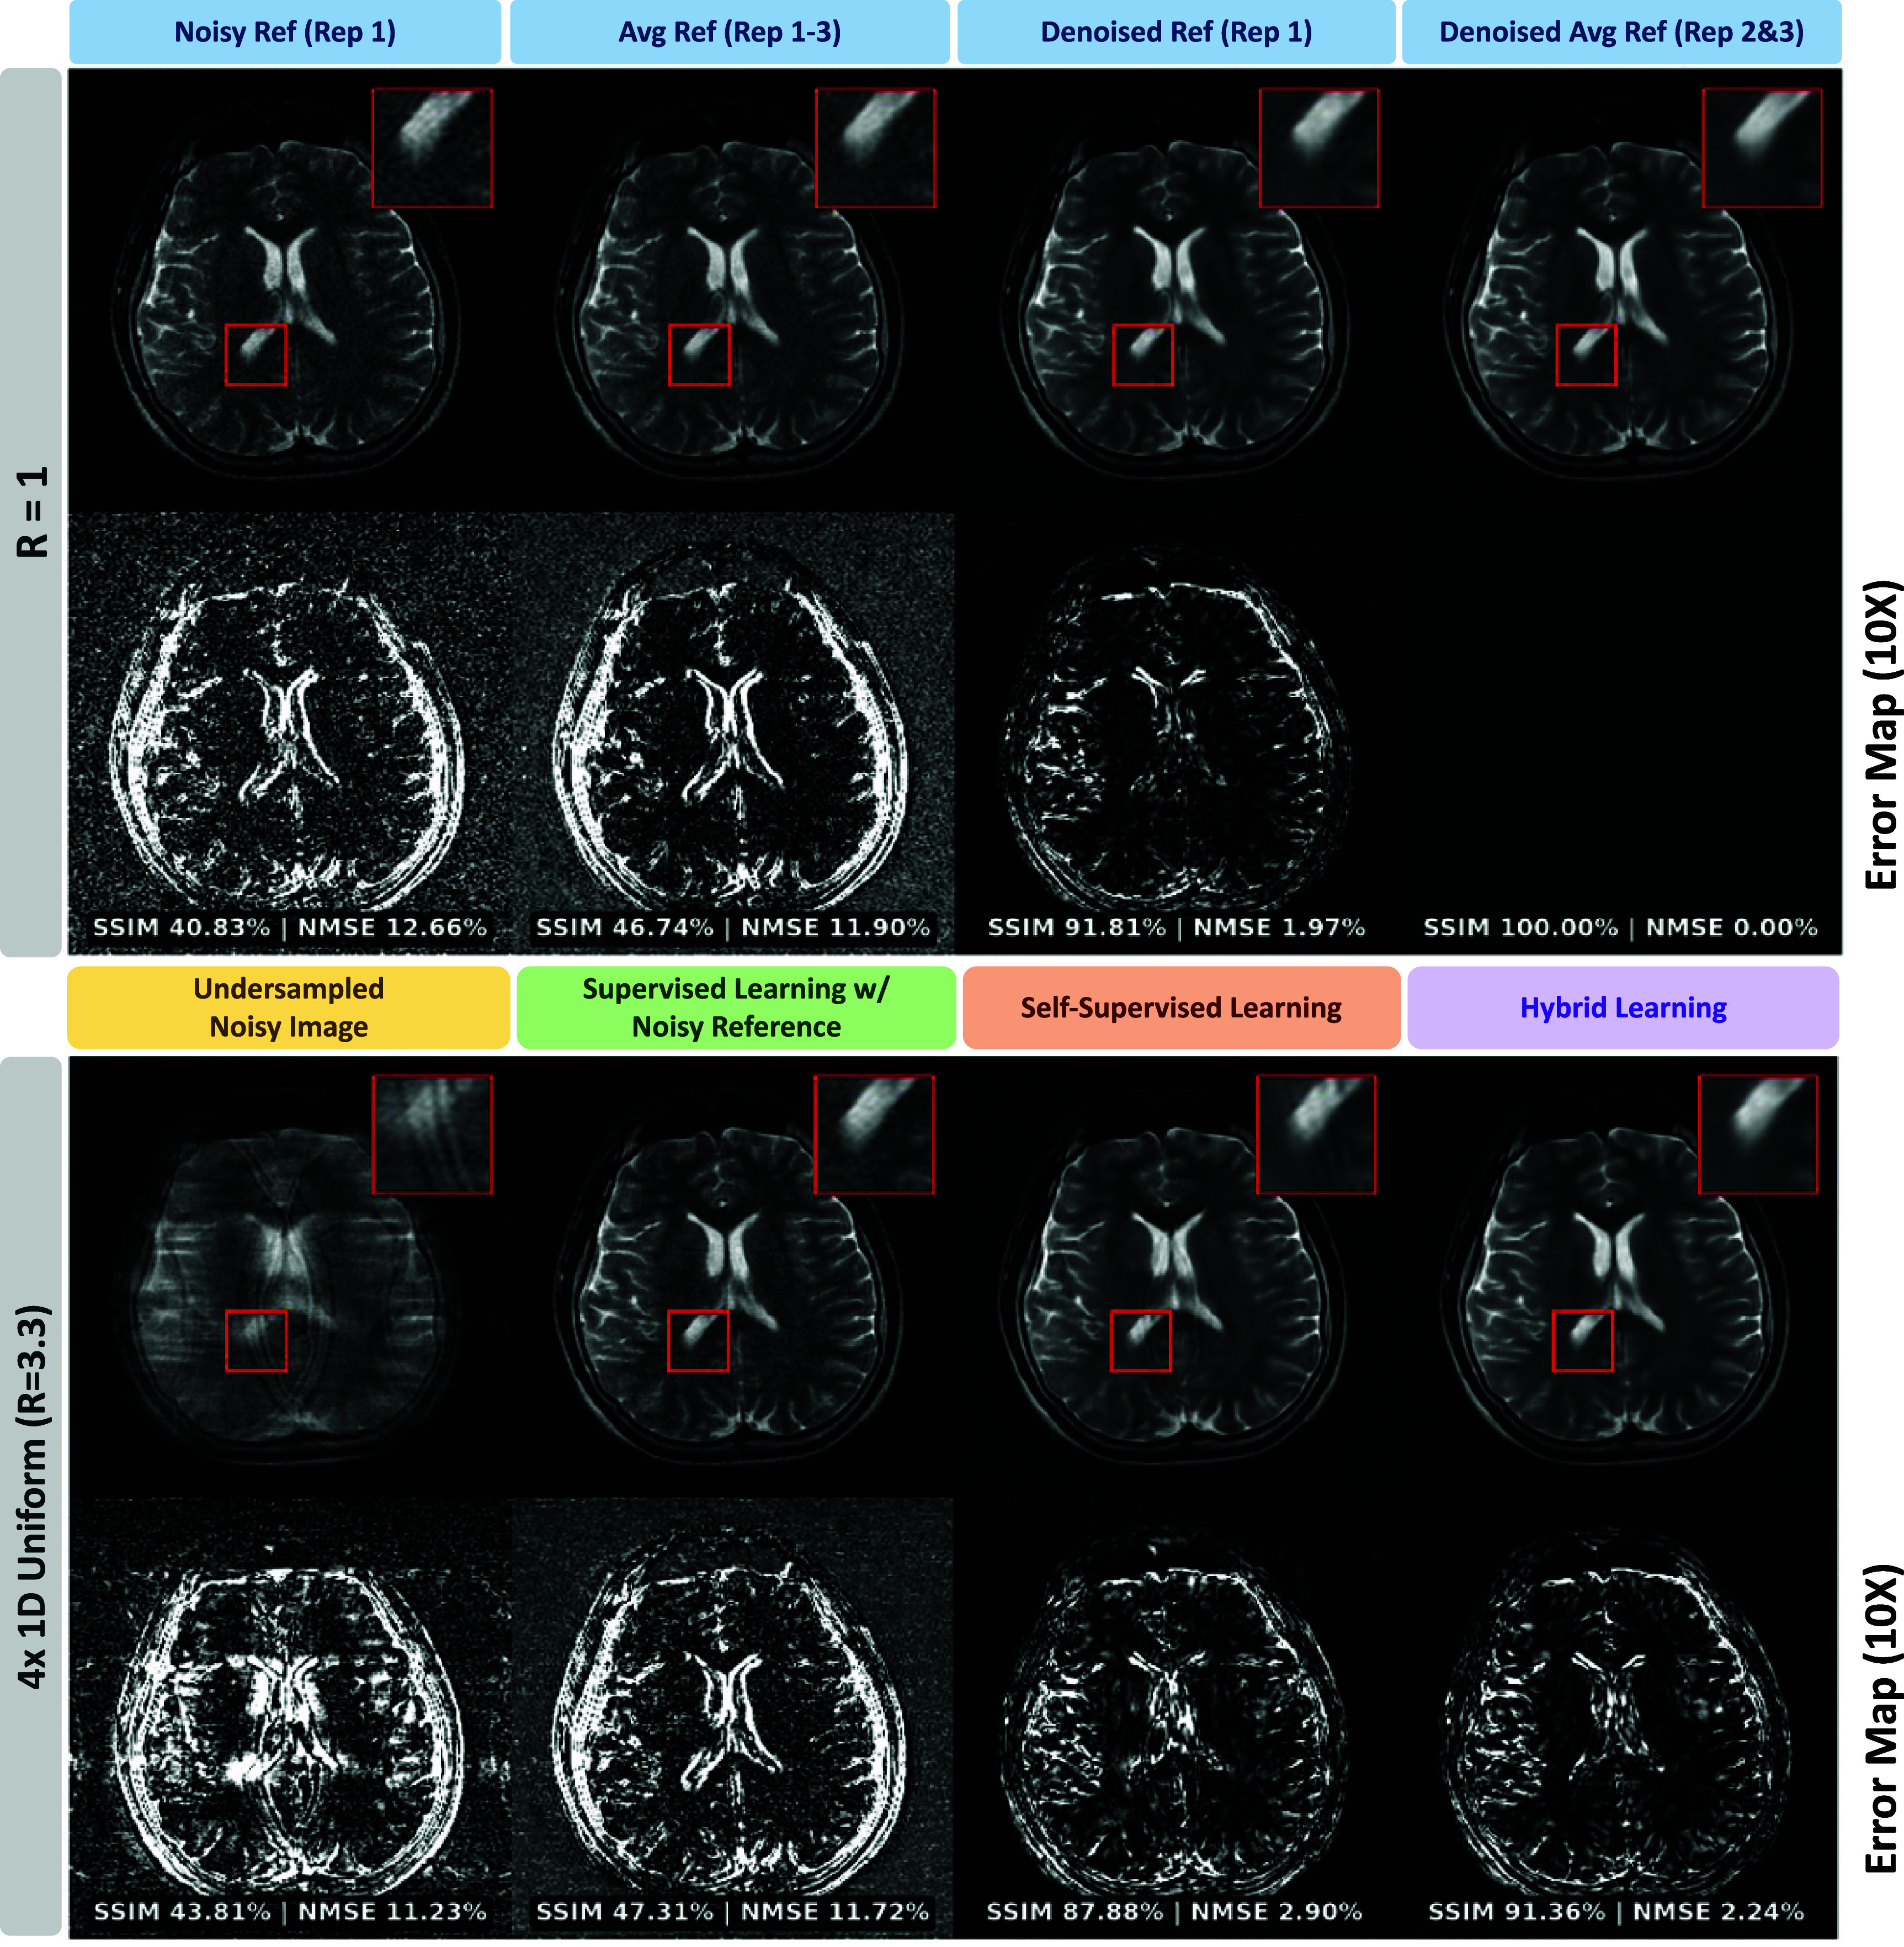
Brain MRI (0.3 T), Cartesian sampling at 4-fold 1D undersampling. A representative case from 0.3 T brain MRI with Cartesian sampling, comparing hybrid learning with supervised and self-supervised learning at 4× 1D uniform undersampling (*R* = 3.3). Top row: (i) Noisy Ref (Rep 1)—the original noisy image from the first repetition, (ii) Avg Ref (Rep 1-3)—the averaged image across all three repetitions, (iii) Denoised Ref (Rep 1)—the denoised image from the first repetition, and (iv) Denoised Avg Ref (Rep 2&3)—the denoised averaged image from the second and third repetitions. Regions of interest (ROIs) and 10× difference maps are provided for reference.
